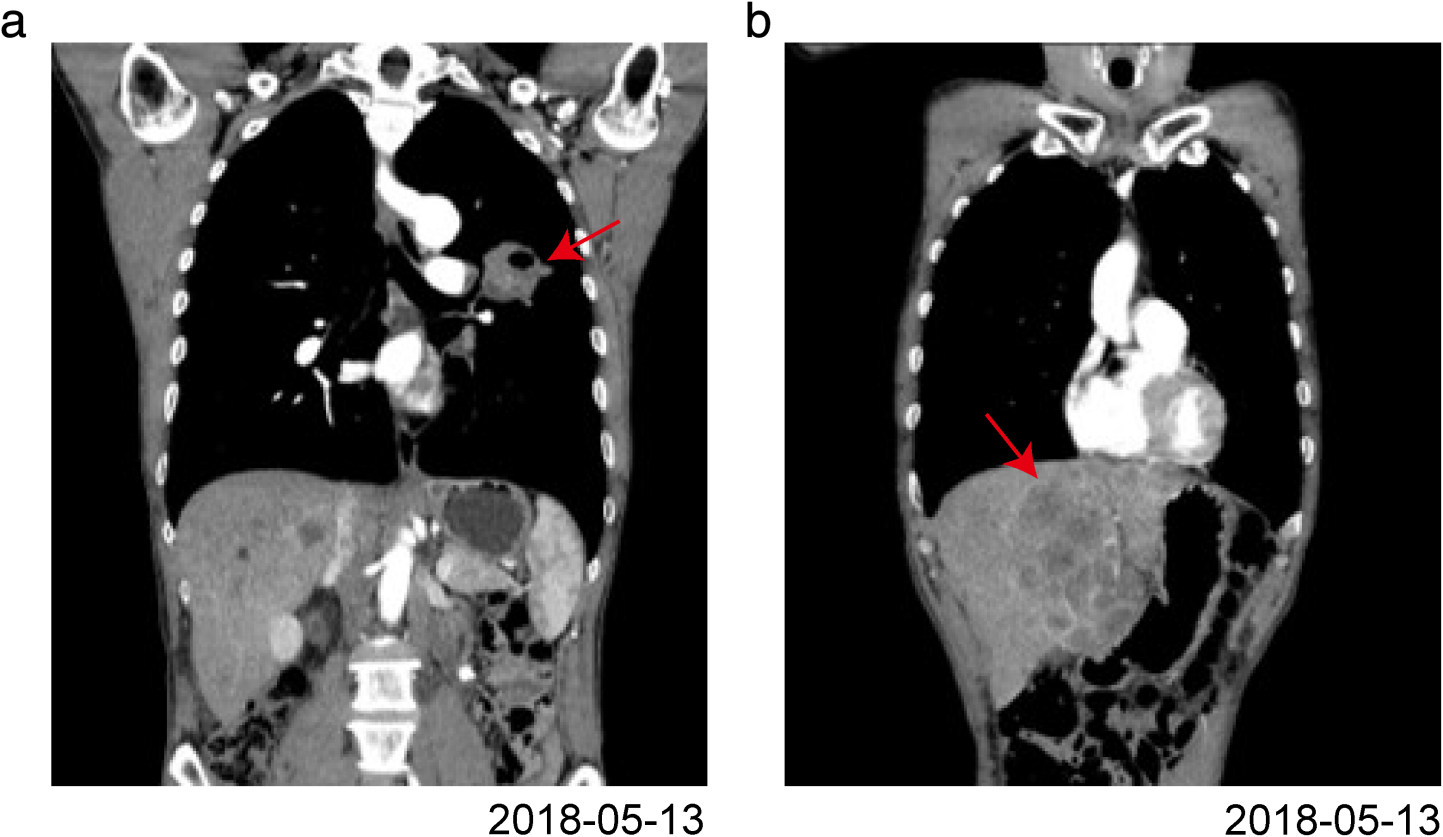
Computed tomography before treatment (a) primary lesion located in the upper left lung with left hilum lymph node metastasis, (b) multiple metastasis seen in the liver.
